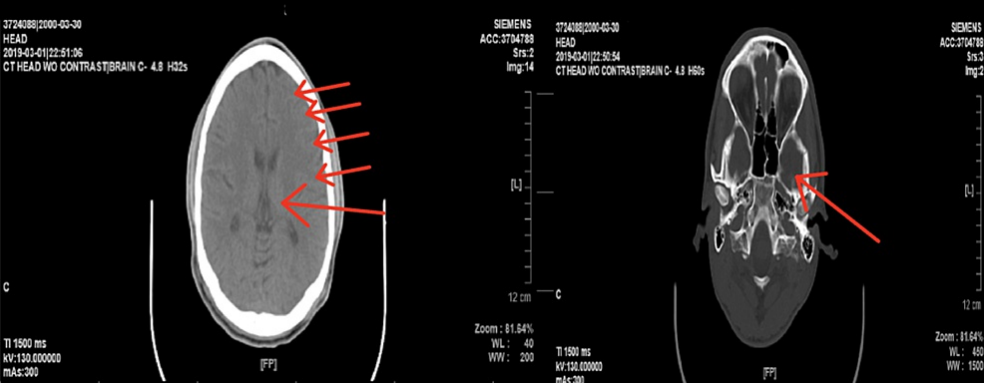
Axial CT without contrast scan of the brain was taken in 2019 (three years after the patient's traumatic brain injury). Small red arrows show no left-sided edema of the brain. A large red arrow indicates no ventricular narrowing.
